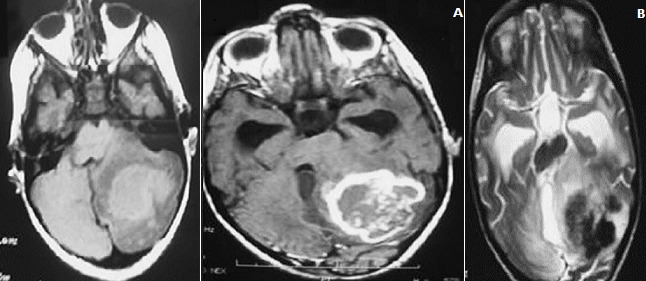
A) brain MRI in axial slices showing a process with isosignal on T1 responsible for a mass effecton vermis and V4 with upstream hydrocephalus; B) enhancing annularly after injection of gadolinium and low signal intensity on T2
